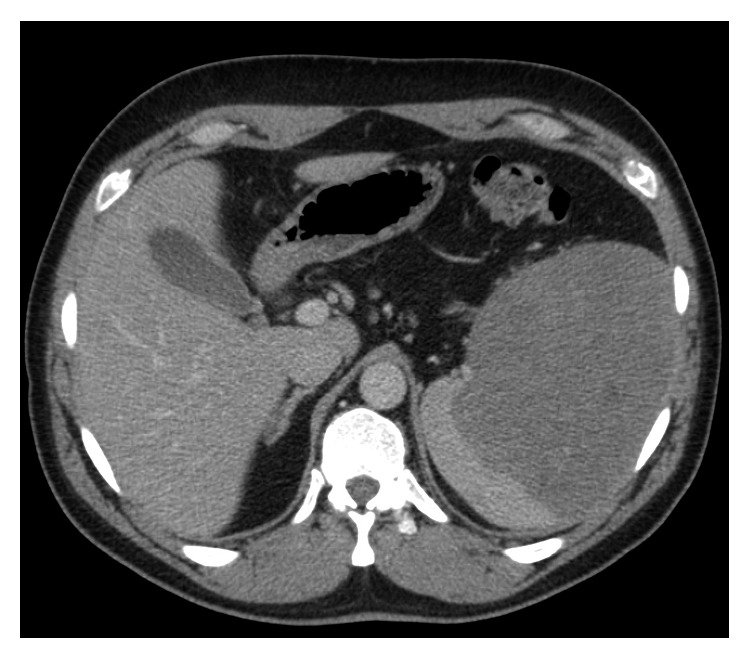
Abdominal CT scan performed before the surgery. Significant spleen enlargement is visible with distinctive area of parenchyma affected by the angiosarcoma.
